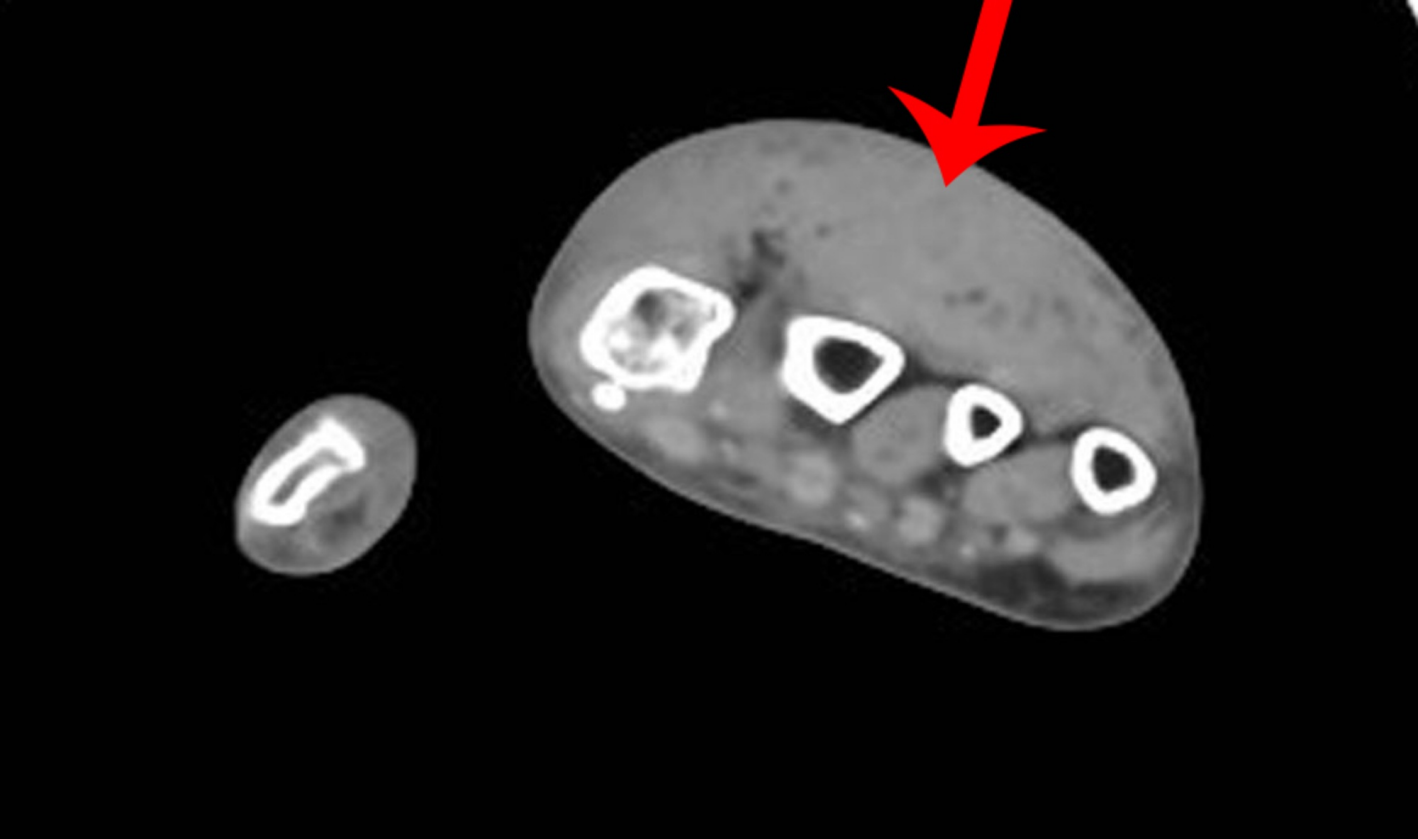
CT scan of the hand (transverse view) CT: computed tomography
